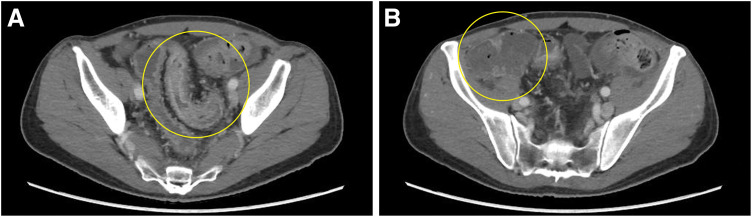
CT findings. (A) Circumferential wall thickening with enhancement extending approximately 10 cm in the sigmoid colon. (B) Marked dilation of the proximal colon was observed.
